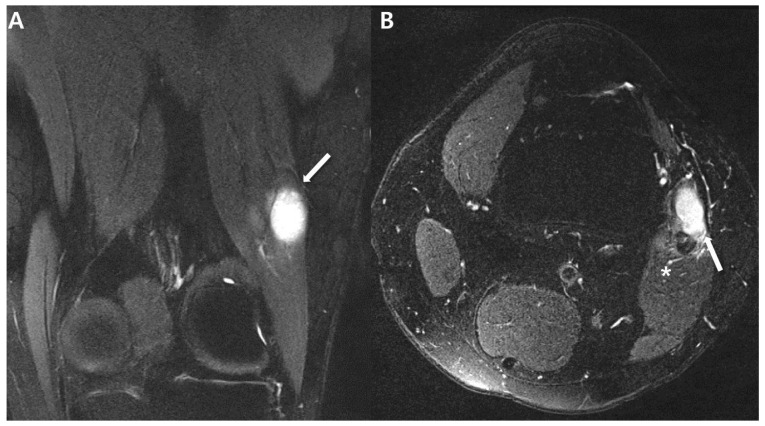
Non-aggressive features on MRI for small (<5 cm) synovial sarcoma of 22-year-old men (biphasic type): (A) Coronal T2-weighted, fat-suppressed image shows about 1.2 × 1.7 × 1.9 cm sized lobulated mass (arrow) in the lateral aspect of Lt knee. (B) Axial T2-weighted. fat-suppressed image shows a mass (arrow) with homogenously bright signal intensity, abutting biceps femoris (*) with adjacent signal change.
